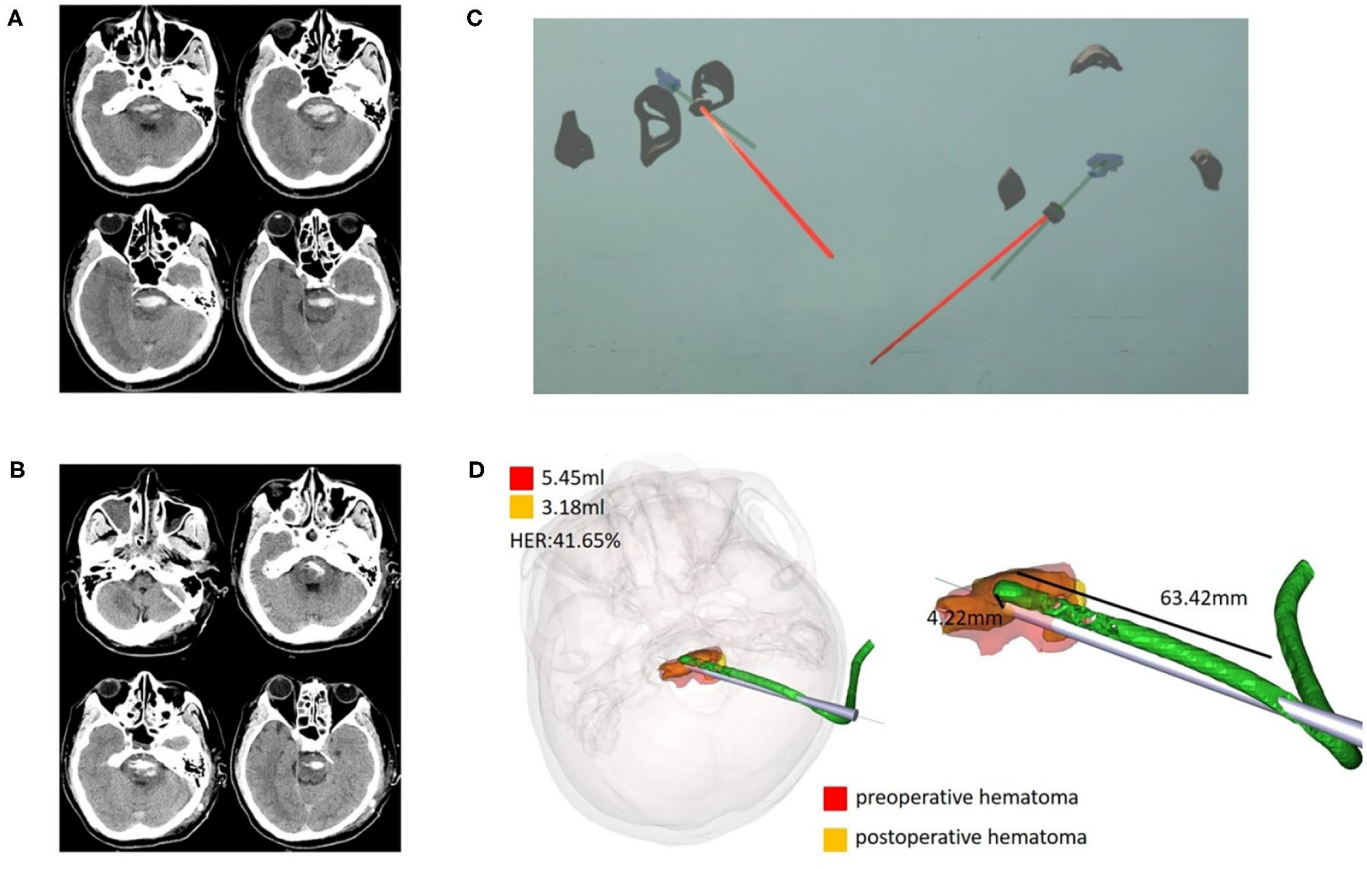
Case 3, a 37-year-old male patient diagnosed with HICH in the brainstem. (A) Preoperative CT showed HICH in the brainstem. (B) Postoperative follow-up CT. (C) Wearing HoloLens, the sagittal and horizontal planes were adjusted for puncture through the ear and nose locations. (D) Fusion of the preoperative and postoperative three-dimensional reconstructions of the hematoma. The preoperative hematoma volume was 5.45 ml, the postoperative hematoma volume was 3.18 ml, and the hematoma evacuation rate was 41.65%. The length of the intracranial drainage tube was 63.42 mm, and the deviation in the drainage tube target was 4.22 mm. HER, hematoma evacuation rate.
